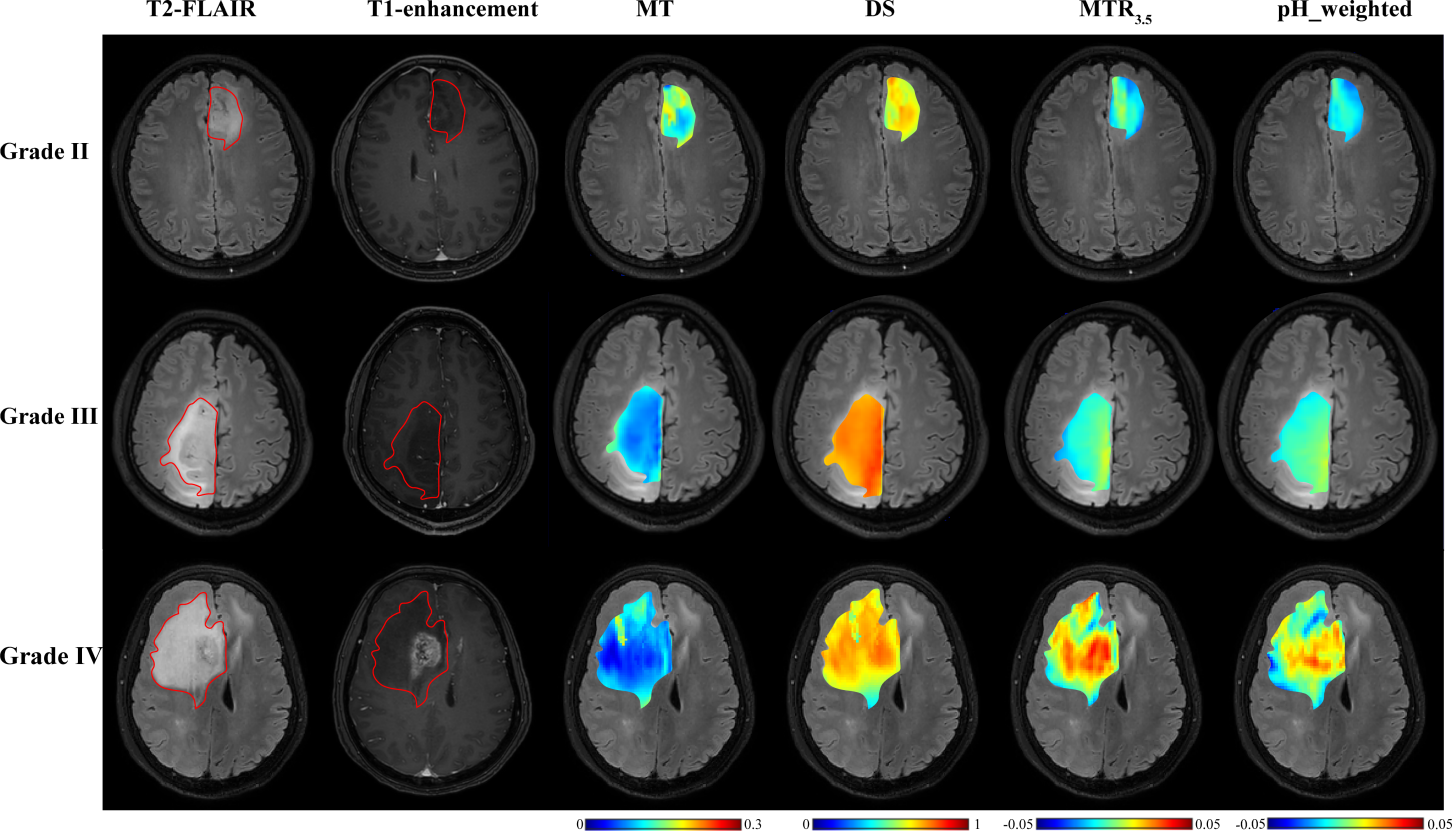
T2-FLAIR, T1-enhancement images and effective CEST derived metric maps of MT, DS, MTR3.5 and pH_weighted for differentiating between one grade II glioma patient (44 years old male), one grade III glioma patient (33 years old male) and one grade IV glioma patient (61 years old male). As glioma grade increases, tumors exhibit higher DS and MTR3.5 signals, lower MT signals, and increased acidity within the tumor region.
